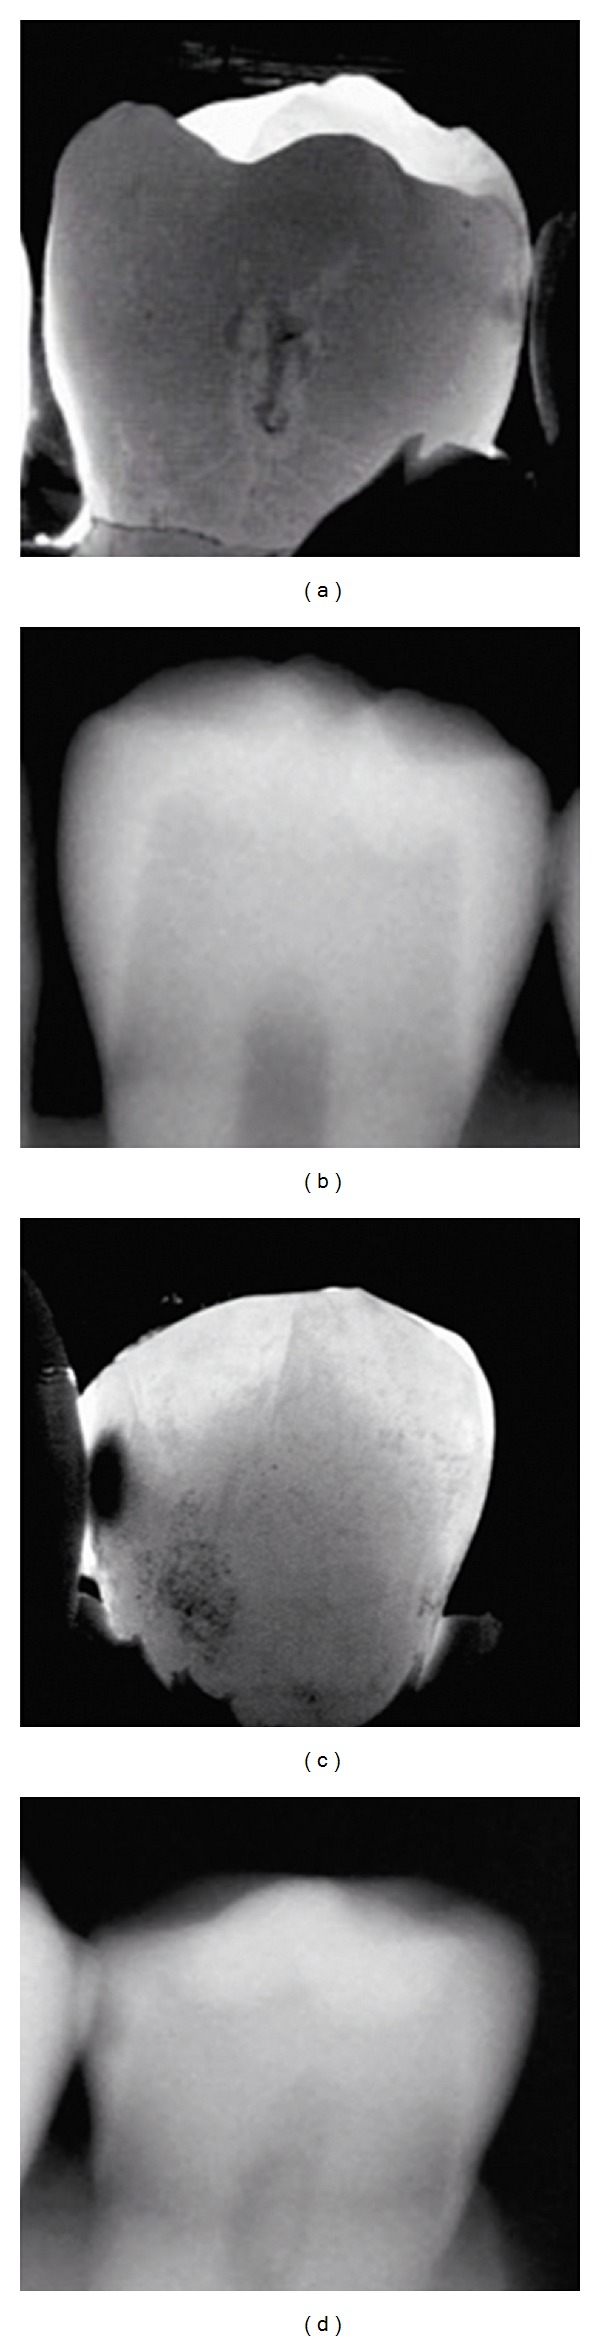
Two sets of images (a) DIFOTI image and (b) digital radiograph of lesion with reference standard score 1. (c) DIFOTI image and (d) digital radiograph of lesion with reference standard score 3.
